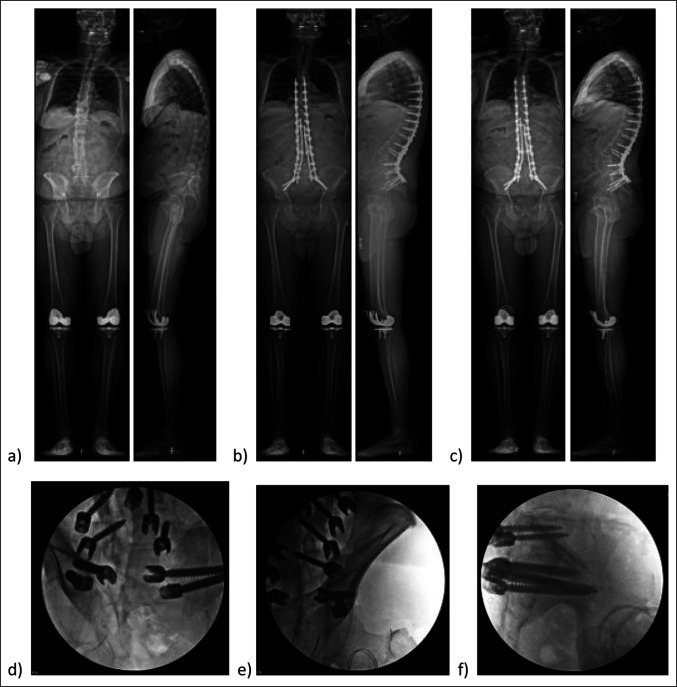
Top: AP and lateral full-length standing radiographs (A) preoperatively, (B) 1 month postoperatively, and (C) 1 year postoperatively. Bottom: Radiographs showing (D) left and (E) right intraoperative teardrop views and (F) lateral view showing placement of the iliac and S2AI screws on each side.
